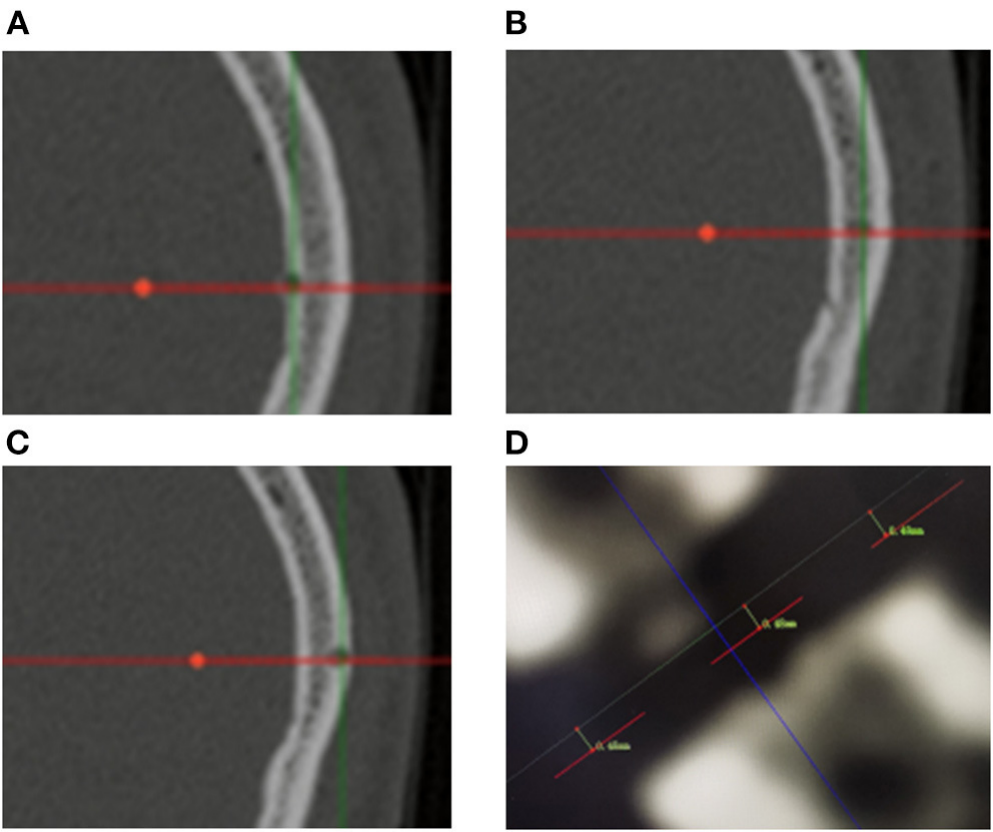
(A–C) Measurement of entry point error based on postoperative CT scanning. The red lines represent the biopsy trajectory planned preoperatively. Bone defects represent the actual biopsy trajectory of the operation. The EPEs are computed as the average of the measurement of the inner part, midpoint and outer part of the cranial bone. (D) The picture shows the measurement results of one of the patients. The EPEs were 0.48, 0.48, and 0.49 mm, respectively.
